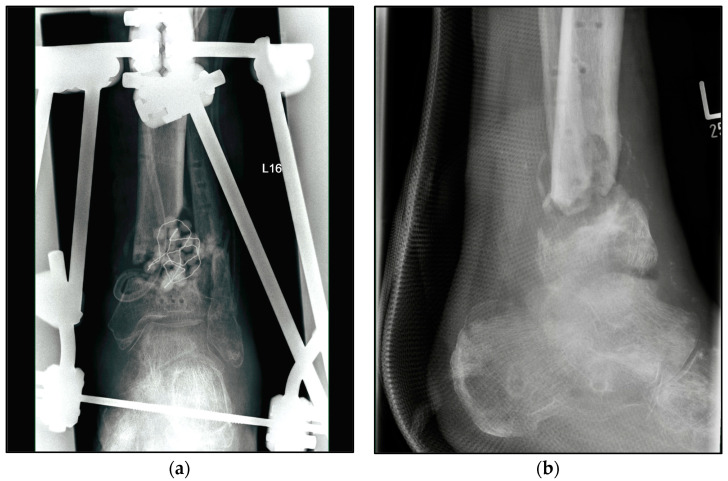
Radiographic findings of the left ankle of a 82-year-old male patient with condition according to open 3° tibial fracture with septic history and treated with external fixator. (a) Anteroposterior view; (b) lateral view.
